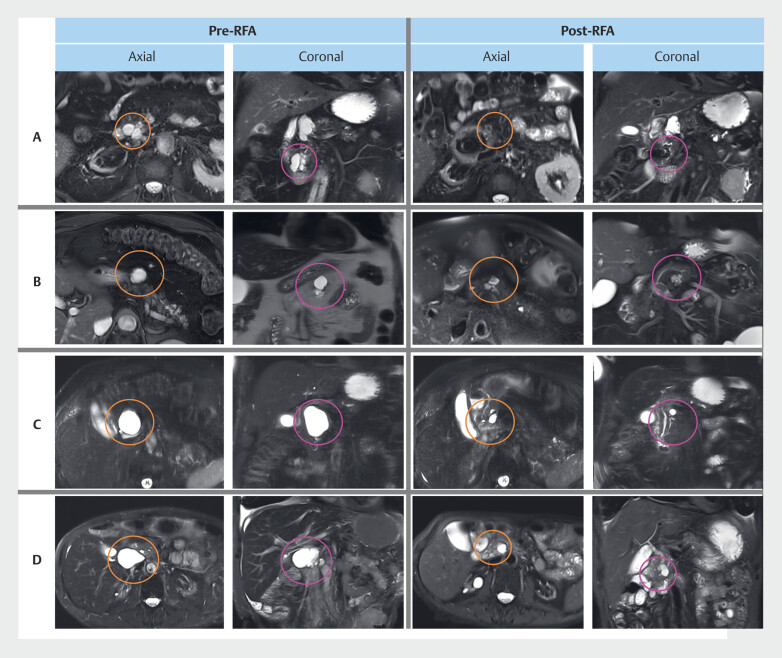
Representative volume reduction of BD-IPMNs assessed with MRI following endoscopic ultrasound-guided radiofrequency ablation (EUS-RFA). Each row represents a single BD-IPMN from a unique participant, with the lesion highlighted in axial images (orange circle) and coronal images (pink circle). BD-IPMN volumes were quantified using region-of-interest (ROI) segmentation and computerized three-dimensional (3D) reconstruction. The respective post-RFA volume reductions for BD-IPMNs in Panels A, B, C, and D, are 97%, 54%, 94%, and 99%, respectively.
